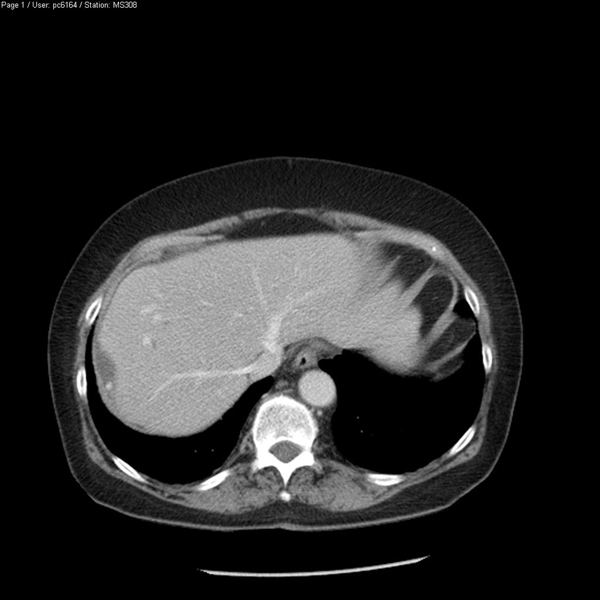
CT demonstrating perihepatic gallstone.
